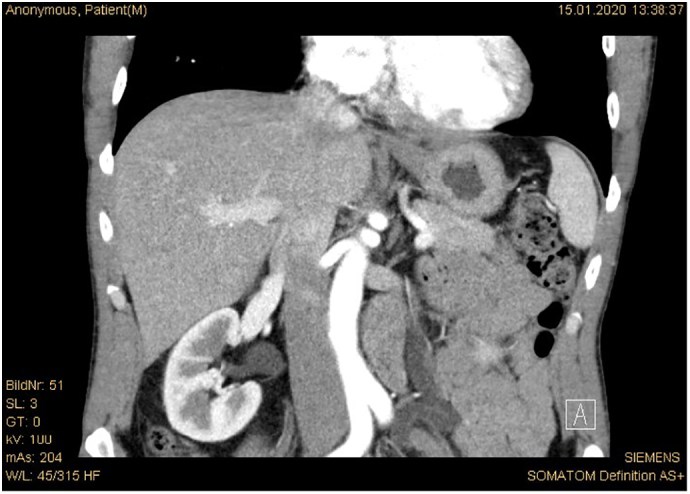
Contrast-enhanced computed tomography scan (arterial phase) showing slightly enlarged common iliac artery with kinking and concomitant secondary hydronephrosis.
